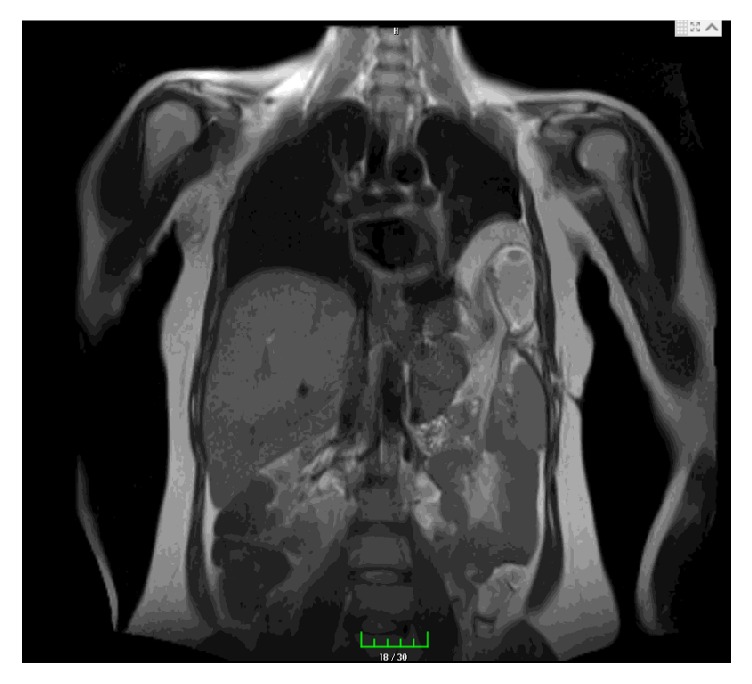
Thoracoabdominal magnetic resonance imaging showing irreducible Bochdalek hernia.
